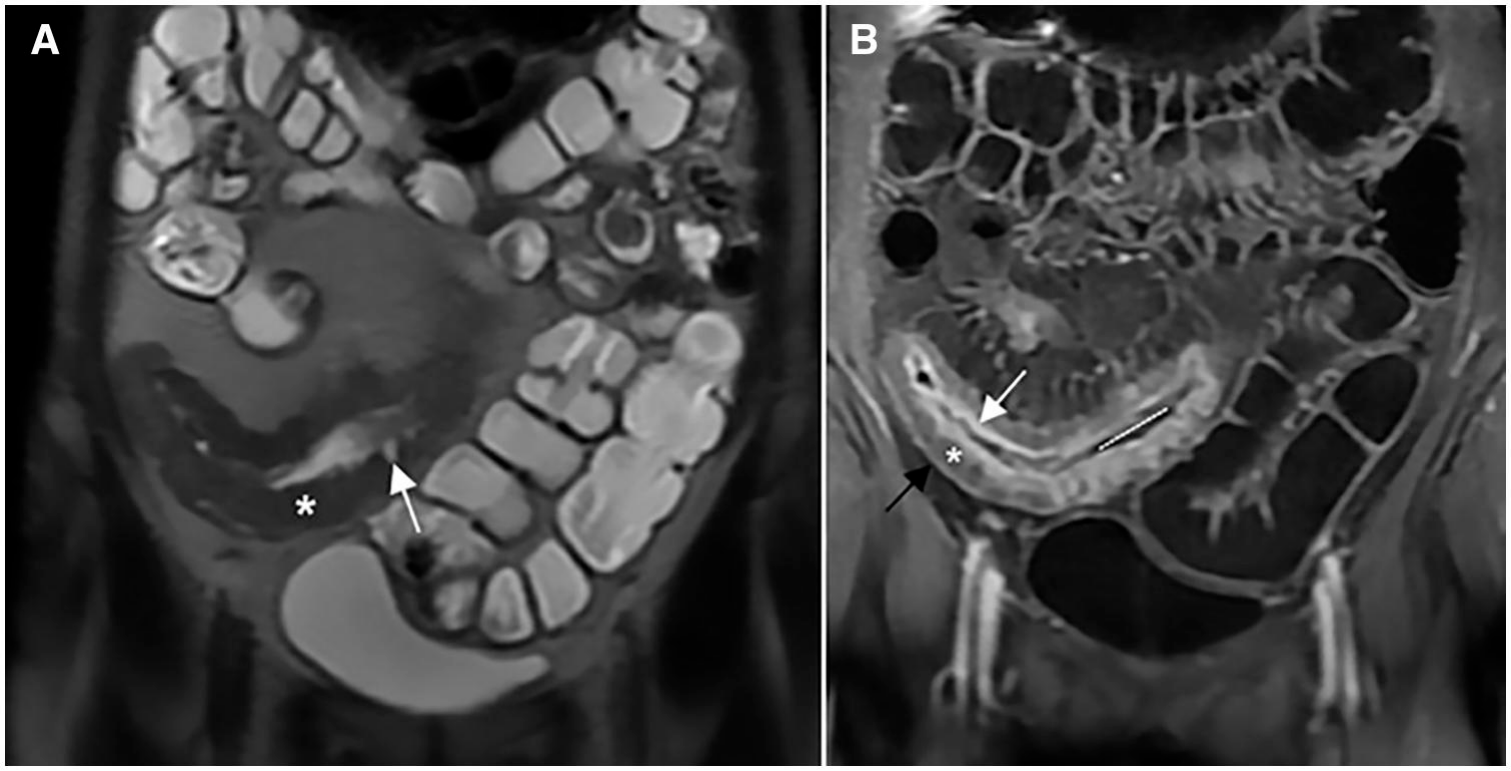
MR-enterography in a patient with known Crohn’s disease: coronal T2-weighted image (A) shows marked thickening of an ileal loop in the right lower abdominal quadrant. Oral biphasic contrast medium (polyetilen-glycole solution) accentuates the signal difference between the lumen and the bowel wall, so it permits to identify wall thickening (white asterisk) and mucosal ulceration (white arrow). The thickness of the small bowel is pathological when it is >3 mm. (B) Coronal T1-weighted image after gadolinium injection shows wall thickening of the ileal loop in the right lower abdominal quadrant with stratified contrast enhancement: the mucosa (white arrow) and the outer layer (the muscle layer and serosa, black arrow) have higher signal due to hyperaemia, while submucosa (asterisk) shows low signal intensity due to oedema. The low signal of the lumen (dashed line) permits us to better appreciate mucosal enhancement. The stratified contrast enhancement is one of the disease activity signs.
